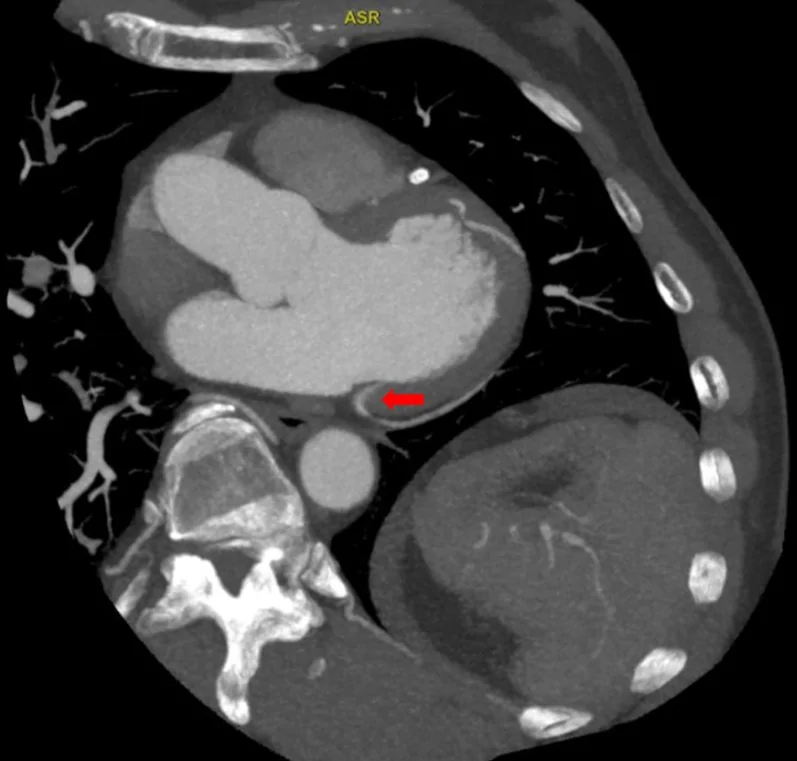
Coronary Computed Tomography Angiography The first diagonal artery fistula inserting into the basal aspect of the left ventricle.
